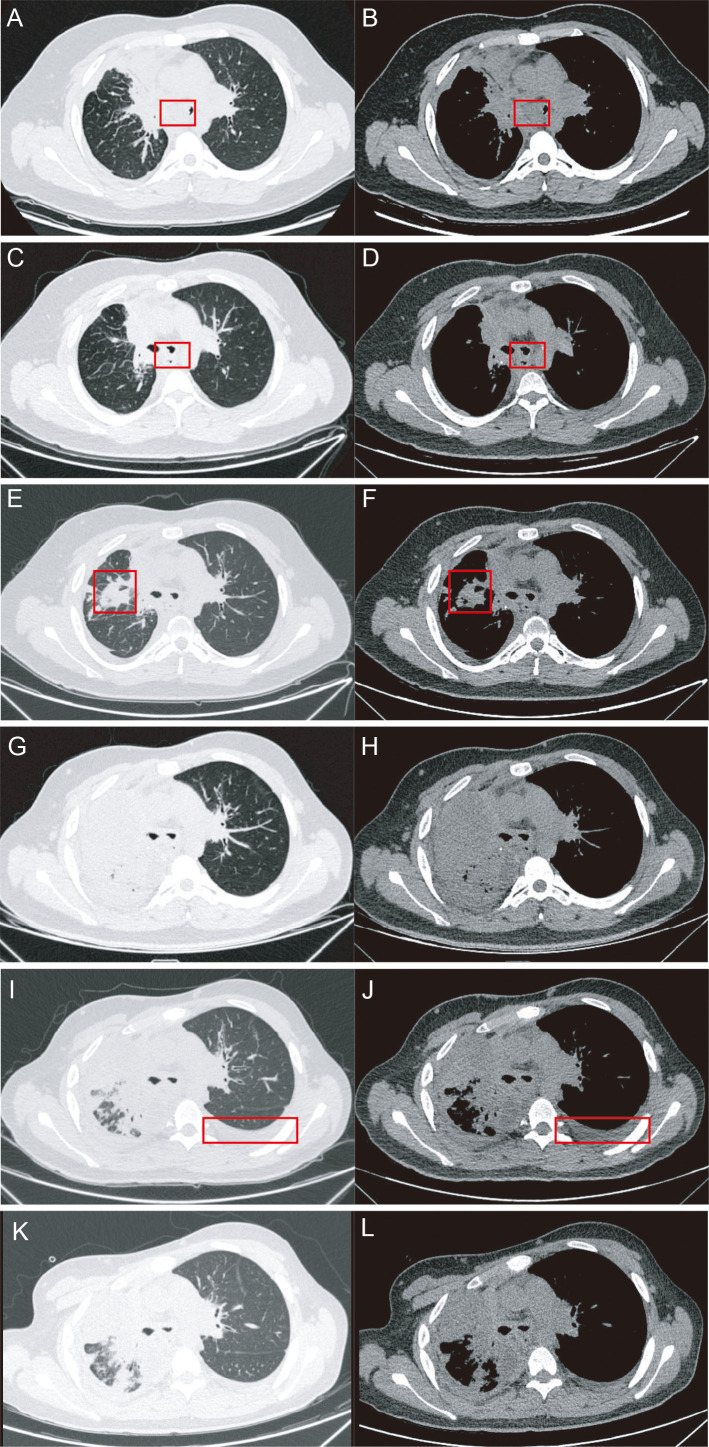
Chest CT images. Before anlotinib plus camrelizumab therapy (A), Lung window. (B), Mediastinal window). 3-cycle reevaluation during anlotinib plus camrelizumab therapy (C), Lung window. (D), Mediastinal window). 5-cycle reevaluation during anlotinib plus camrelizumab therapy (E), Lung window. (F), Mediastinal window). Before gemcitabine therapy (G), Lung window. (H), Mediastinal window). 4-cycle reevaluation during nab-paclitaxel plus camrelizumab therapy (I), Lung window. (J), Mediastinal window). 5-cycle reevaluation during nab-paclitaxel plus camrelizumab therapy (K), Lung window. (L), Mediastinal window).
